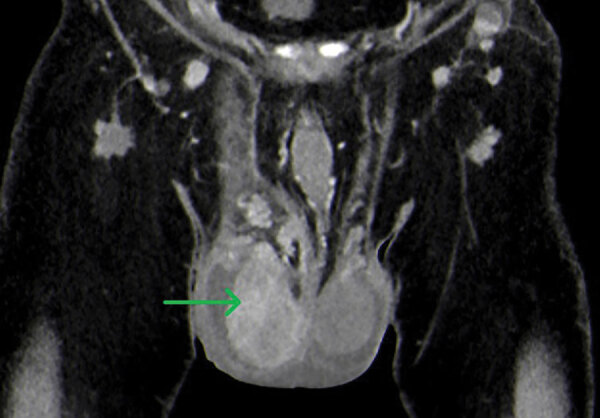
Computed tomography of patient who had human tularemia epididymo-orchitis caused by *Francisella tularensis* subspecies *holartica*, Austria. Coronal image shows the right testicle (arrow) during the arterial phase with hyperperfusion and nonperfused areas (abscess).
